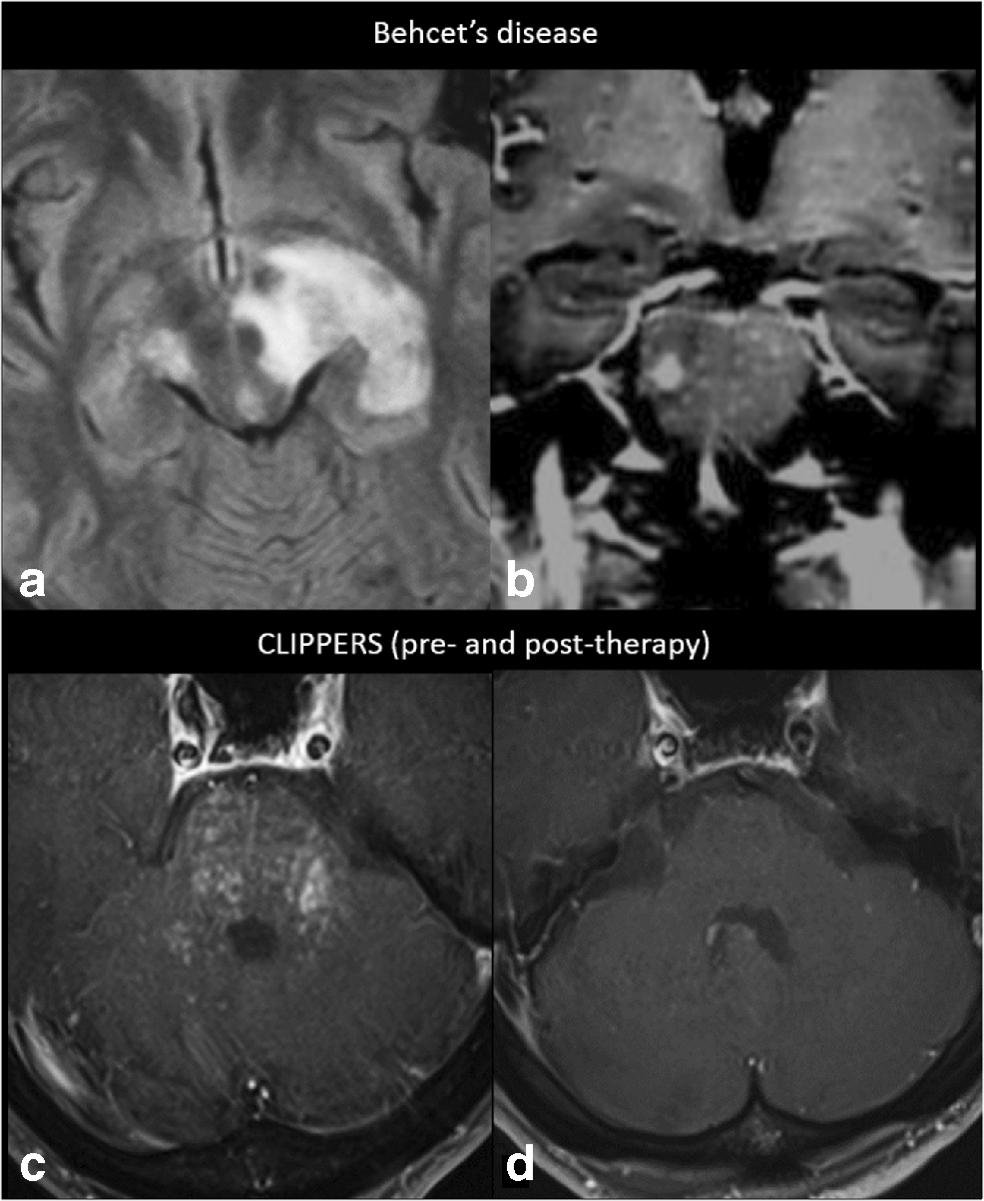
Preferentially infratentorial localisation. A 35-year-old male diagnosed with Behçet’s disease (small intestine biopsy proven) shows in a axial FLAIR MRI at the level of the crus cerebri a heterogeneous left mesodiencephalic lesion with oedema, typically sparing the red nucleus, and in b coronal post-contrast T1-weighted MRI at the level of the pons miliary enhancement and one larger nodule. c Axial post-contrast T1-weighted MRI of a 55-year-old male diagnosed with CLIPPERS, showing miliary-enhancing nodules and slight linear enhancement along the PVS in the pons, dentate nuclei and spreading through the cerebellum and a d complete resolution of the infratentorial lesions after corticosteroid therapy
